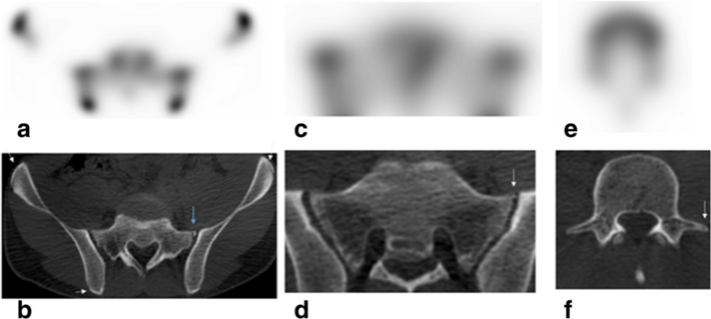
Normal appearance of bone scan and CT scan in a 17-year-old male with mild symmetric uptake in the secondary ossification centers (apophyses). Normal prominent uptake is seen associated with iliac crest apophysis (secondary ossification center) which starts ossifying in this age range and fuses later (until 25 years old). a Axial SPECT image shows symmetric uptake seen in the iliac crest apophyses and subtle uptake associated with small left S1 transverse process apophysis (*blue arrow*) seen on the corresponding CT (b). c Axial SPECT image shows no significantly increased uptake in the small anterior costal epiphysis seen on axial CT image in the expected location (arrow in d). e Axial SPECT image of a lumbar vertebra with no significant uptake seen on axial CT image at the transverse process apophysis on the left (*arrow* on f). Familiarization with the normal appearance and location of the secondary ossification centers is important in the interpretation of back pain to avoid confusion with a small avulsion fractures
